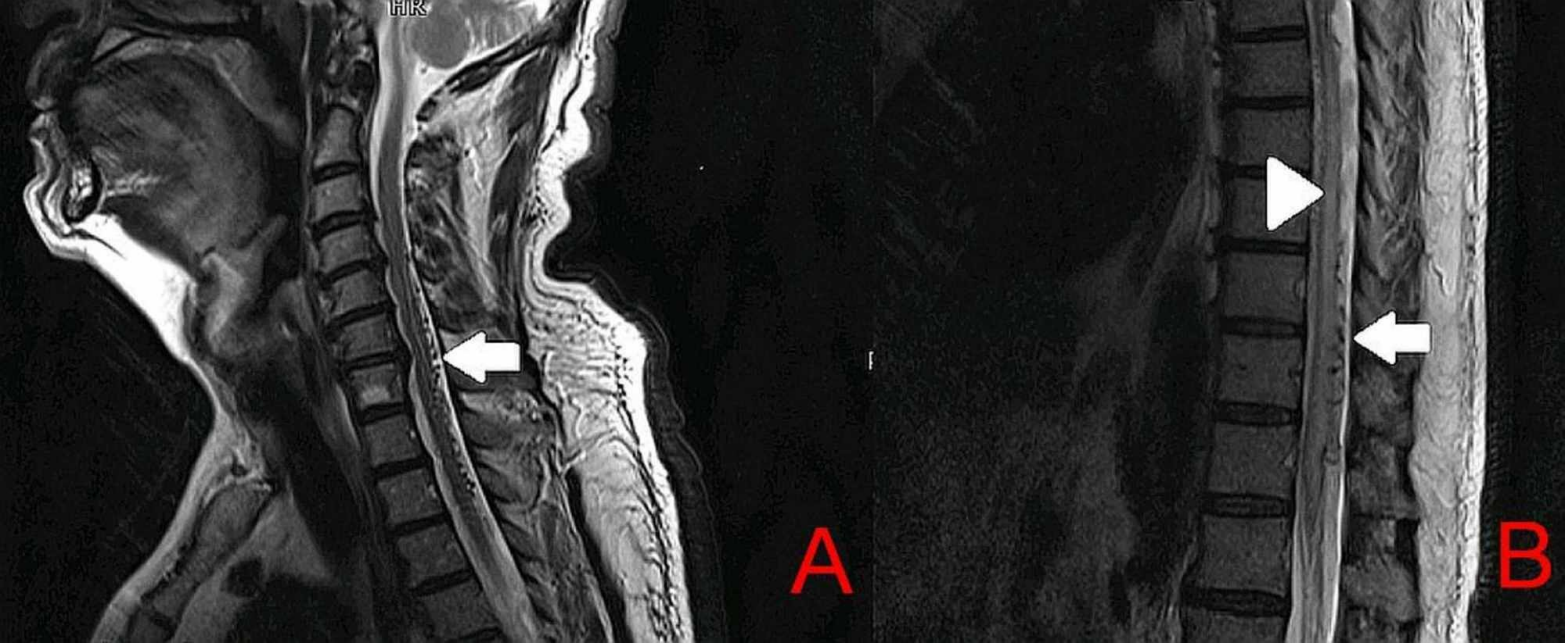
T2 MRI of the cervical spine (A) and thoracic spine (B) White arrows indicate vessel flow voids dorsally. Arrowhead indicates T2 hyperintense signals and cord edema MRI - magnetic resonance imaging
